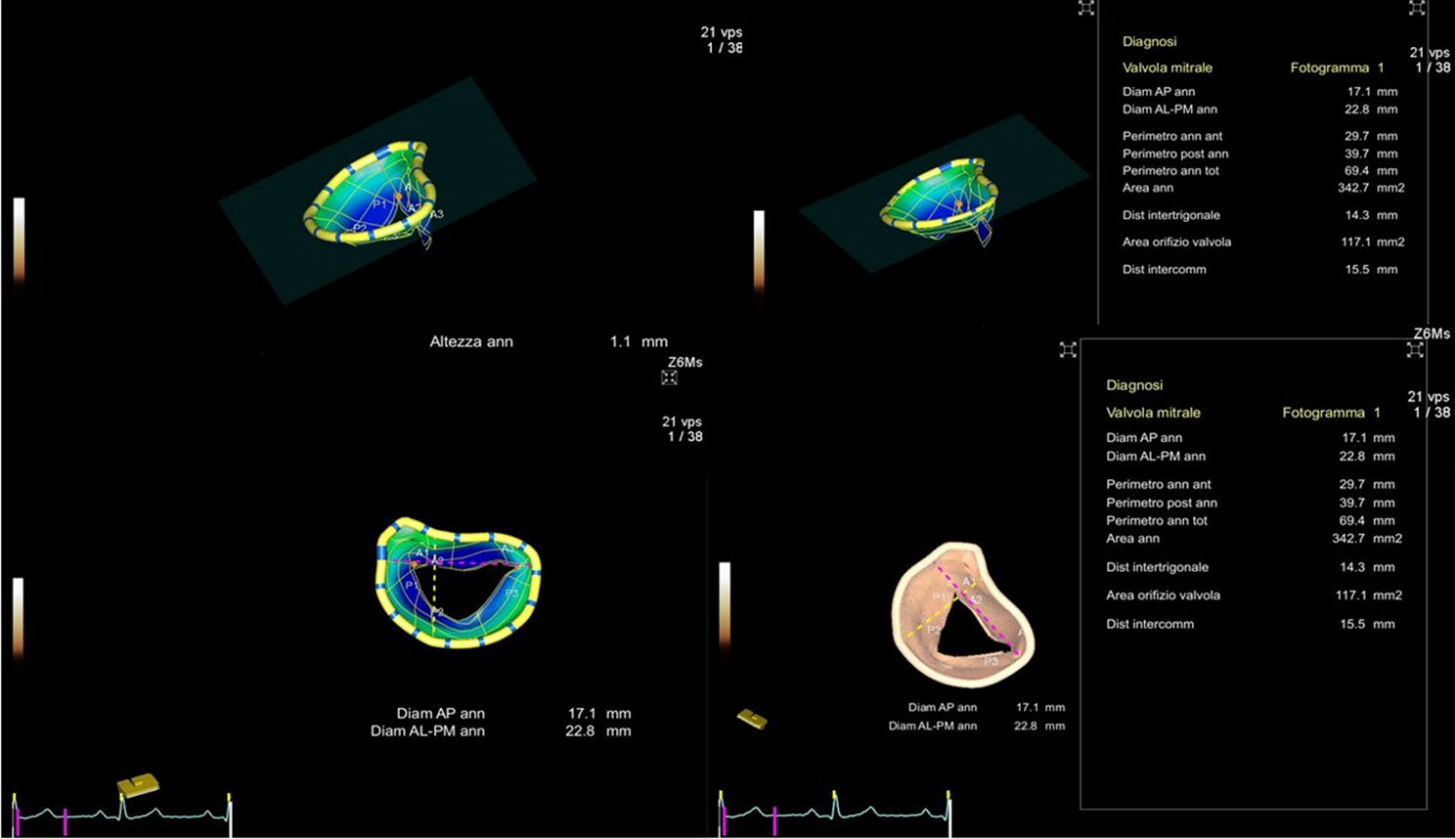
3-Dimensional echocardiographic reconstruction and assessment of mitral annulus with measurement of mitral annulus dynamics at end-systole and end-diastole
